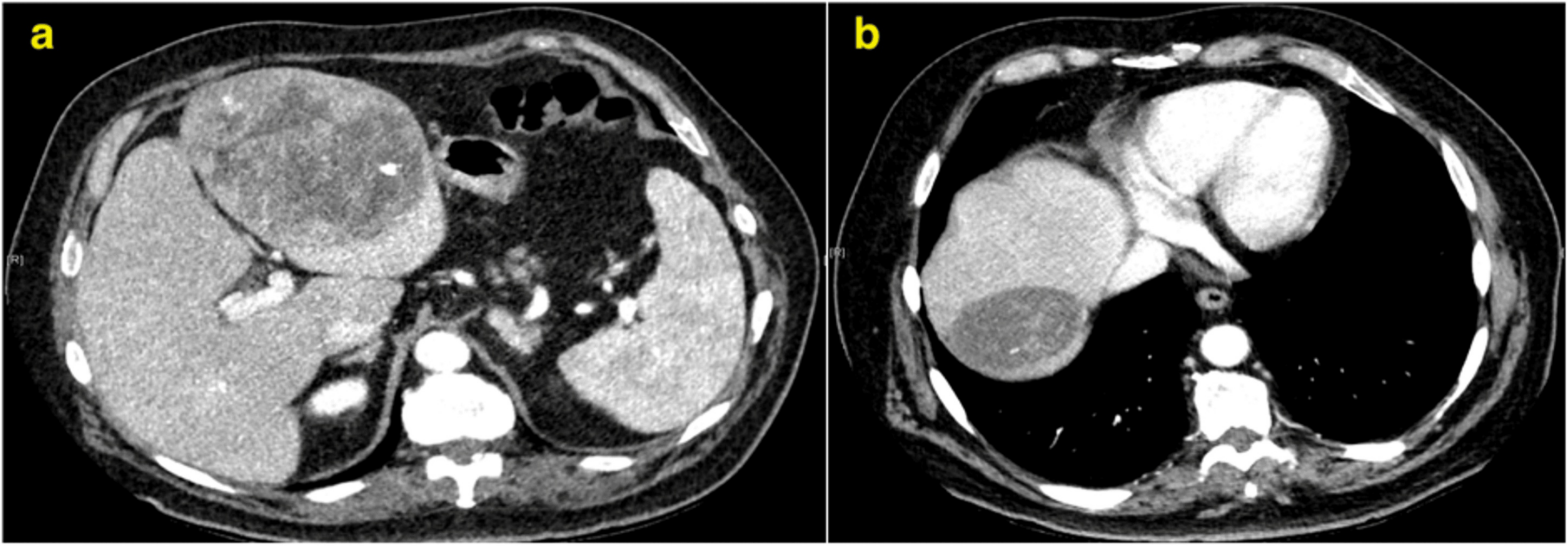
Triple phase computed tomography (CT) axial view of the liver demonstrating a well-defined heterogenous mass in the left lobe with intense arterial enhancement and no washout with areas of haemorrhage suspicious for a ruptured hepatocellular carcinoma (HCC) (Figure 1a). Lesion in right lobe of liver, more well-defined, heterogenous with focal calcification, later identified as a hydatid cyst (Figure 1b).
